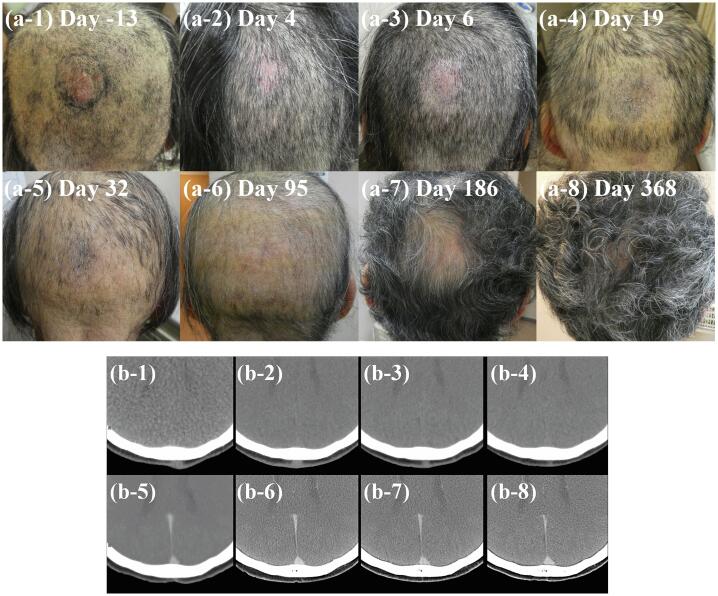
Time course changes in Patient 2 tumor before and after Boron Neutron Capture Therapy (BNCT). (a) Clinical photos and (b) computed tomography scans obtained before treatment start (day 13) and on treatment days 4, 6, 19, 32, 95, 186, and 368. The patient achieved PR and CR on days 4 and 19, respectively, according to the RECIST 1.1 criteria.
